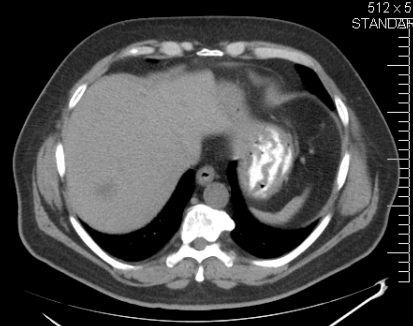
Computed tomography scan of abdomen, showing a hypodense nodule in the right lobe of the liver.
